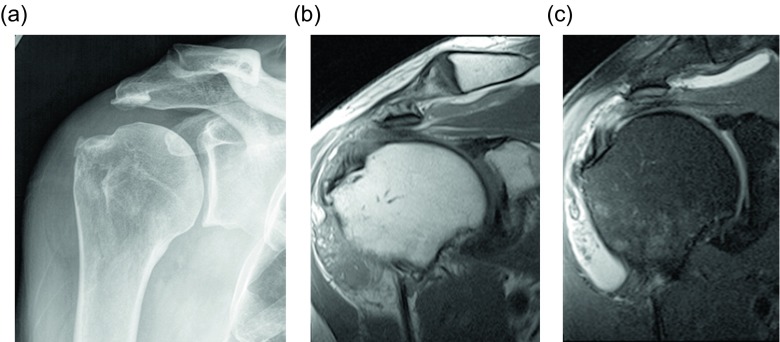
Lipoma arborescens: 70-year-old man with chronic shoulder pain. Frontal radiograph (a) shows a subacromial-subdeltoid effusion. Coronal T1W (b) and T2W FS (c) images demonstrates villous lipomatous proliferation of the synovium outlined by bursal fluid.
